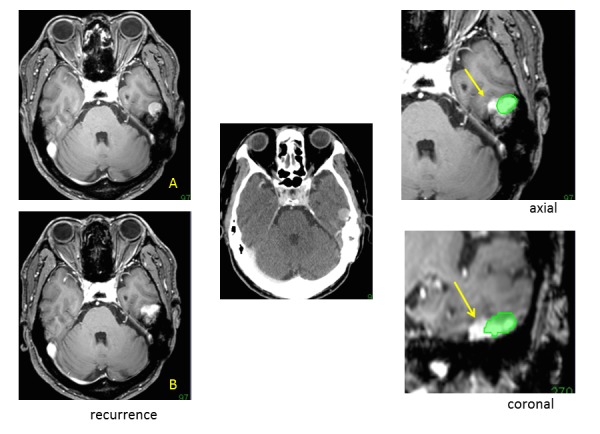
Detection of marginal recurrence. A follow-up MR image after treatment showing recurrence (B) compared with the image before treatment (A). Image fusion using thin-sliced CT (based on the image from radiosurgery, center) enabled us to detect the treated part of the tumor (green) and the recurrent tumor from the medial margin of the tumor (arrows) on identical axial and coronal images (right). The treated part decreased in volume, as is shown in follow-up MR images fused with the image before radiosurgery.
